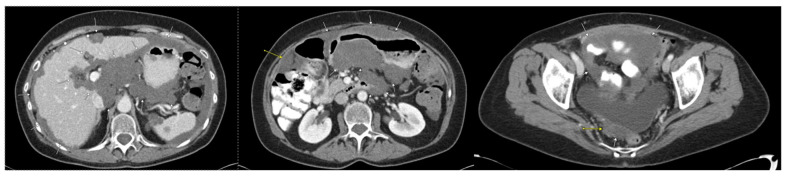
First CT scan of abdomen/pelvis after initial CRS/HIPEC to show disease progression. Arrows indicate diffuse and enlarged mucinous peritoneal metastases and demonstrate interval increase in tumor burden.
